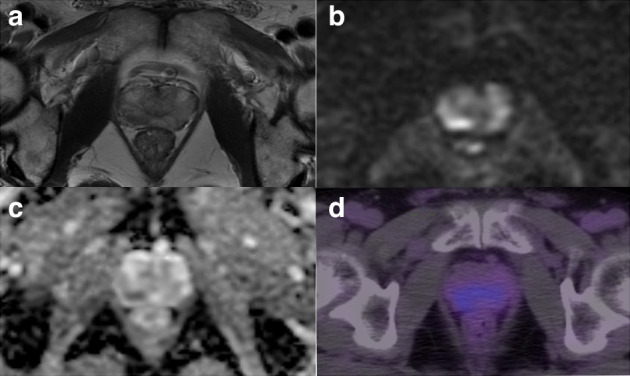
A PI-RADS 4 lesion on mpMRI was negative on ^18^F-PSMA-1007 PET/CT (SUVmax 4.5) and confirmed to be prostate cancer on biopsy. (a) T2-weighted image, (b) diffusion-weighted image, (c) apparent diffusion coefficient map, and (d) ^18^F-PSMA-1007 PET/CT images.
